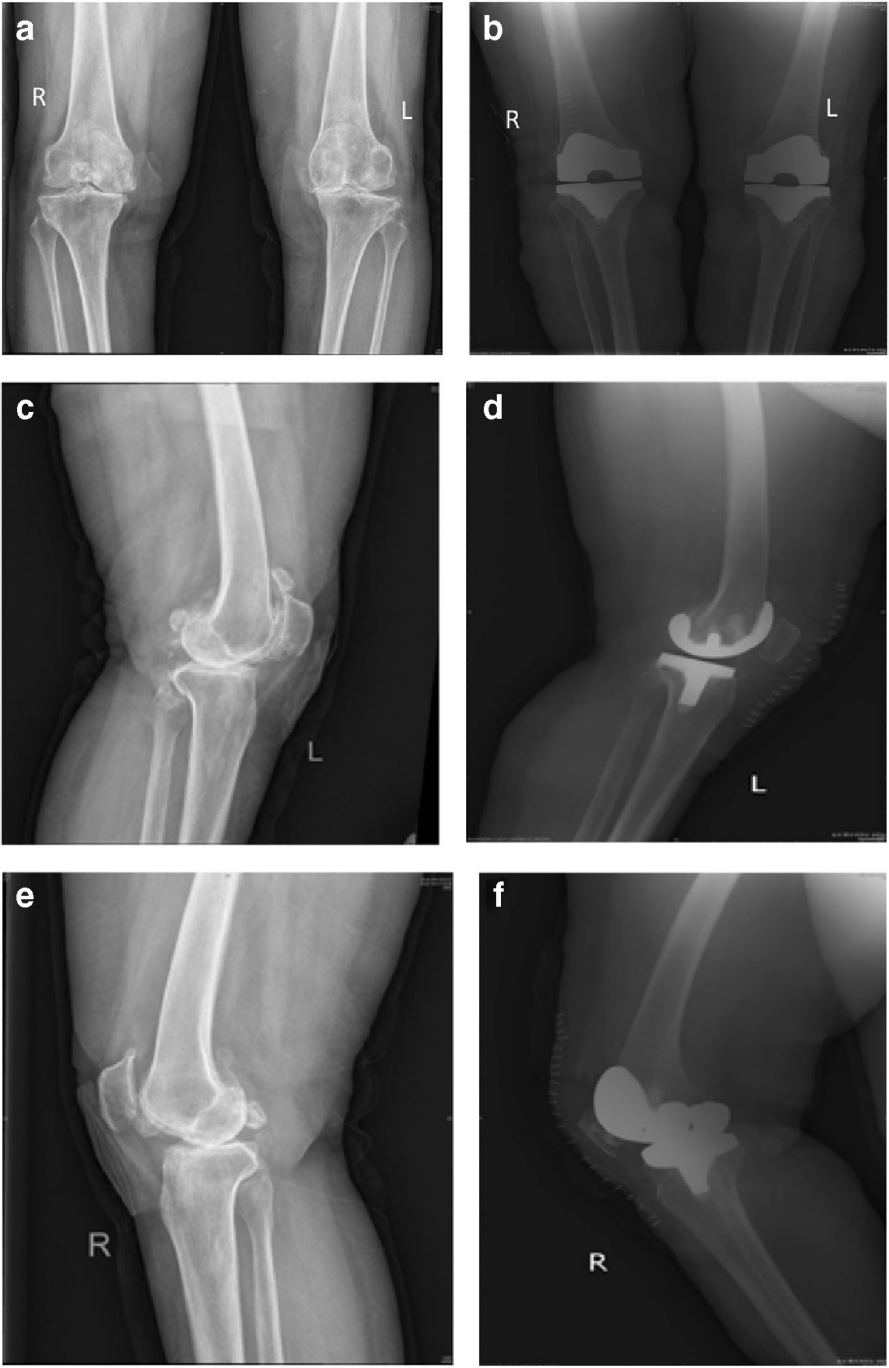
Pre-operative (1 week) and post-operative (3 days) X-ray examinations of a 68-year-old woman with bilateral osteoarthritis. Left: non-resurfaced patella. Right: resurfaced patella. a Anteroposterior position 1 week before operation. b Anteroposterior position 3 days after operation. c Lateral position of left knee joint 1 week before operation d Lateral position of left knee joint 3 days after operation (non-resurfaced). e Lateral position of right knee joint 1 week before operation. f Lateral position of right knee joint 3 days after operation (resurfaced)
